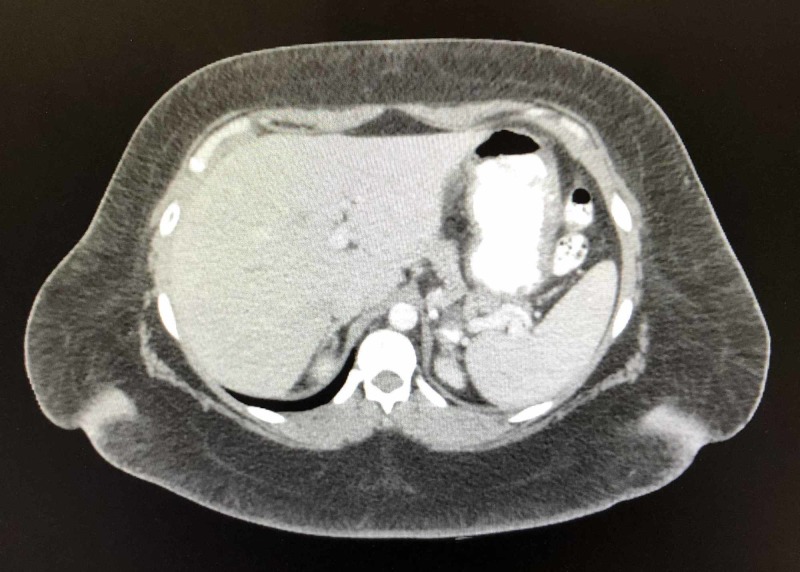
Axial preoperative CT scan was unremarkable.
